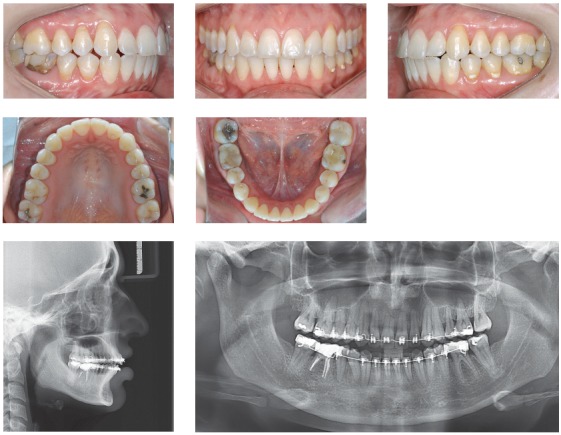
Initial records: intraoral photographs, lateral cephalogram and panoramic radiograph.
